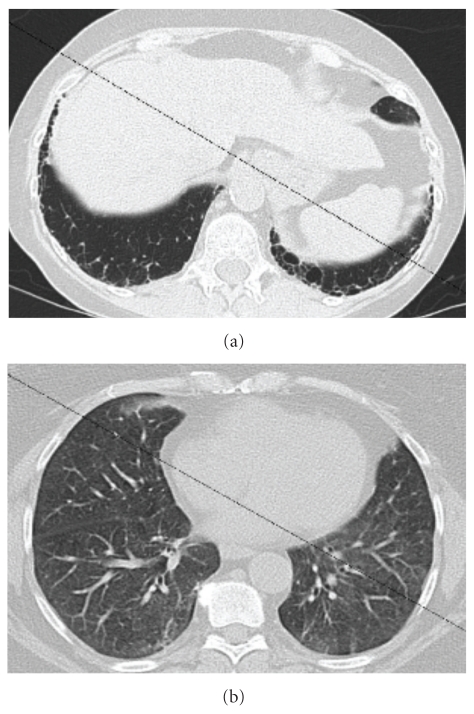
HRCT demonstrating predominantly reticular findings (a) as well as ground glass changes (b).
